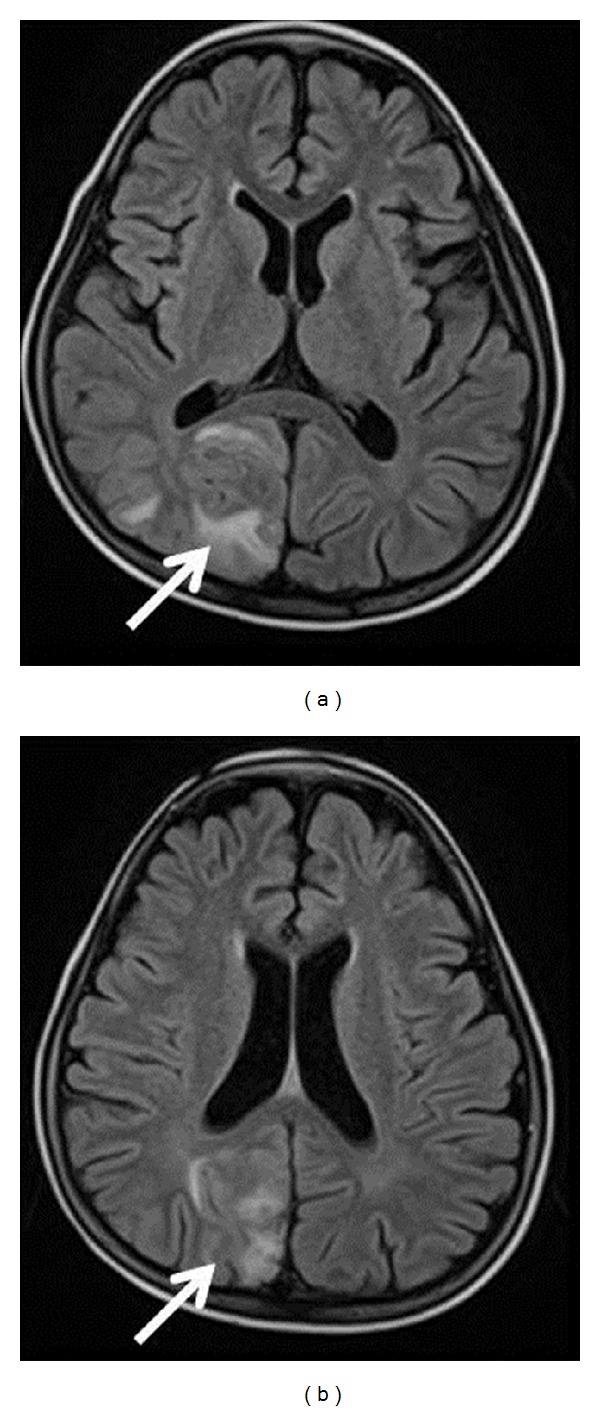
FLAIR brain MR images of a 12-year-old girl with sporadic paraganglioma admitted to our Institution with headache and seizures. Unilateral right occipitoparietal lobe vasogenic edema (arrows), typical of PRES, is well depicted after a severe hypertensive crisis. PRES = Posterior reversible encephalopathy syndrome.
